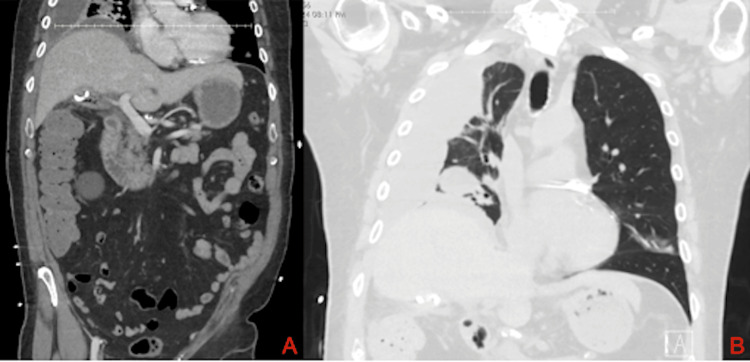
Preoperative cross-sectional imaging. (A) CT scan of the abdomen demonstrating the percutaneous cholecystostomy tube traversing the liver and curling within the contracted gallbladder with small components of gas. A partially visualized right-sided loculated pleural effusion is also seen. (B) CT of the thorax following PCT exchange again demonstrates the loculated pleural effusion, along with visualization of the PCT within the gallbladder. PCT: percutaneous cholecystostomy tube.
